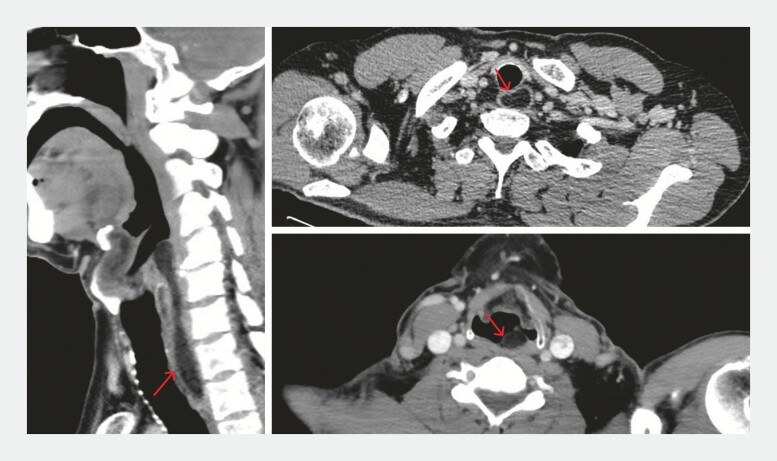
Contrast-enhanced CT showed a mass with fat density, without significant contrast enhancement.
